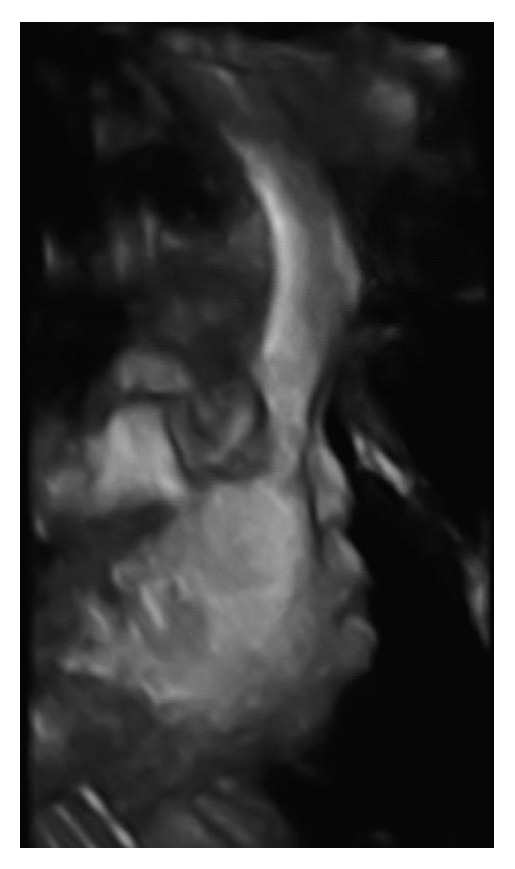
3D ultrasound at 21 weeks shows striking nasal hypoplasia.
